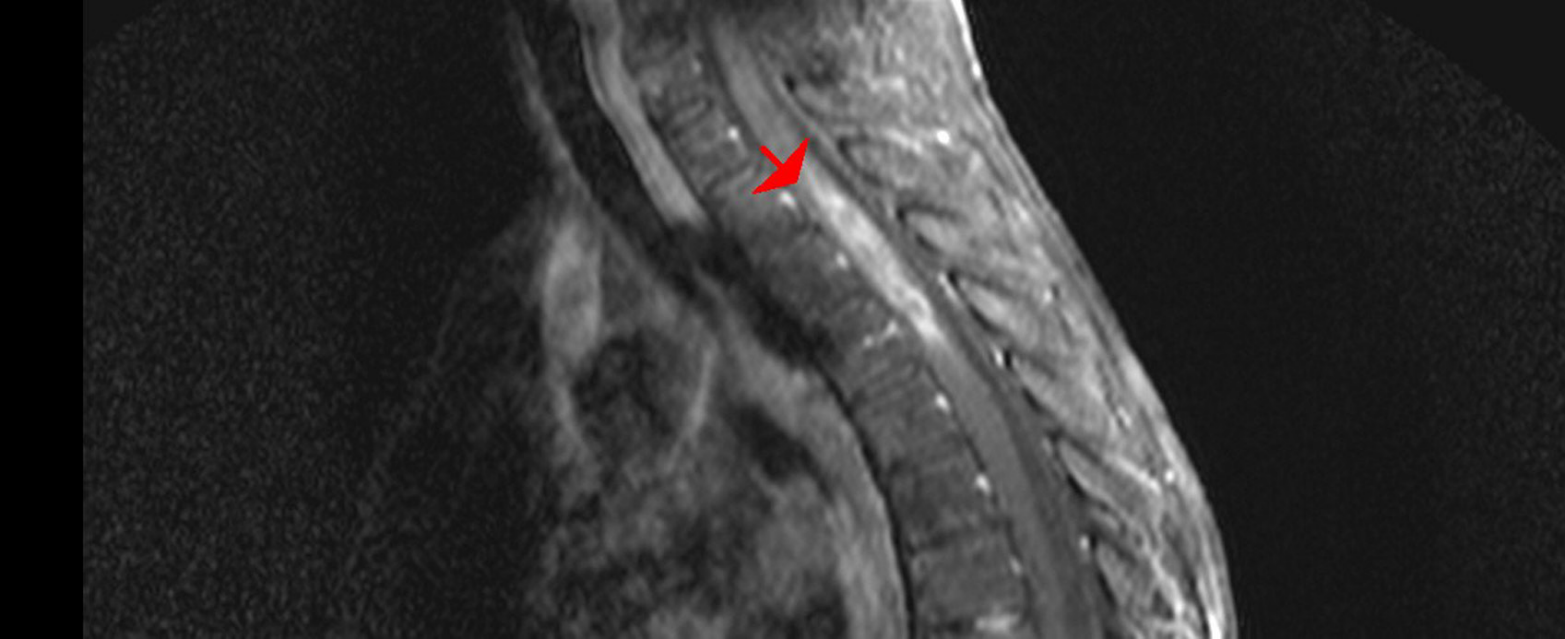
Thoracic MRI post GAD. Sagittal T1 Gd + spinal MRI shows an intradural intramedullary tumor at T2, T3 level (hyper signal lesion or avidenhancement).
